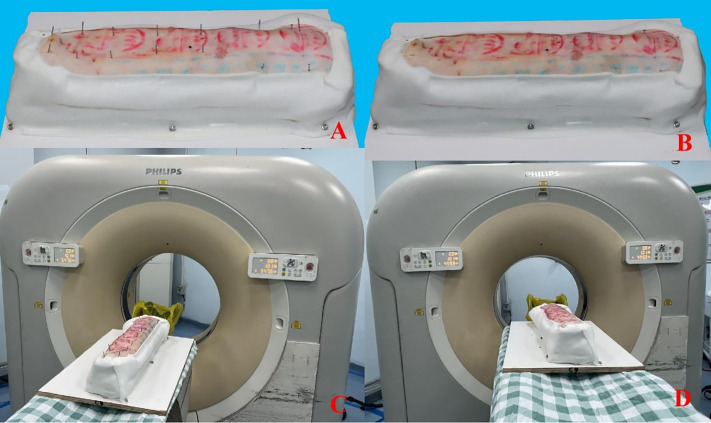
Postoperative CT Scan. A Experimental model with the needle in place; B Experimental model without the needle; C CT scan of the experimental model with the needle in place; D CT scan of the experimental model without the needle.
